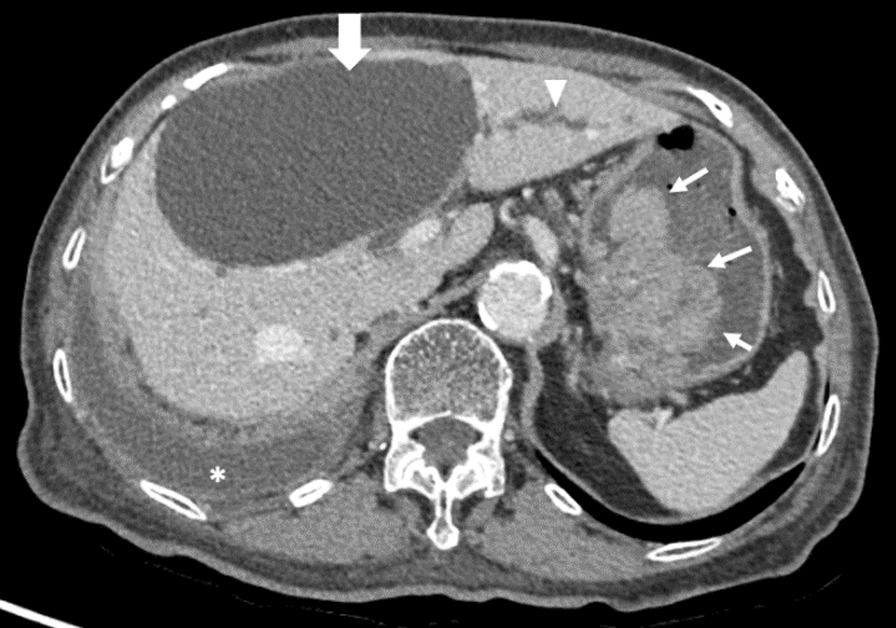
Venous phase of computed tomography at upper abdomen level. A 6.9 × 4.0 × 3.4 cm enhancing polypoid lesion in the stomach involving lesser curvature and gastric fundus (thin arrow), a large hepatic cyst (thick arrow) with adjacent intrahepatic duct dilation (arrow head), and right pleural effusion (*) were demonstrated
